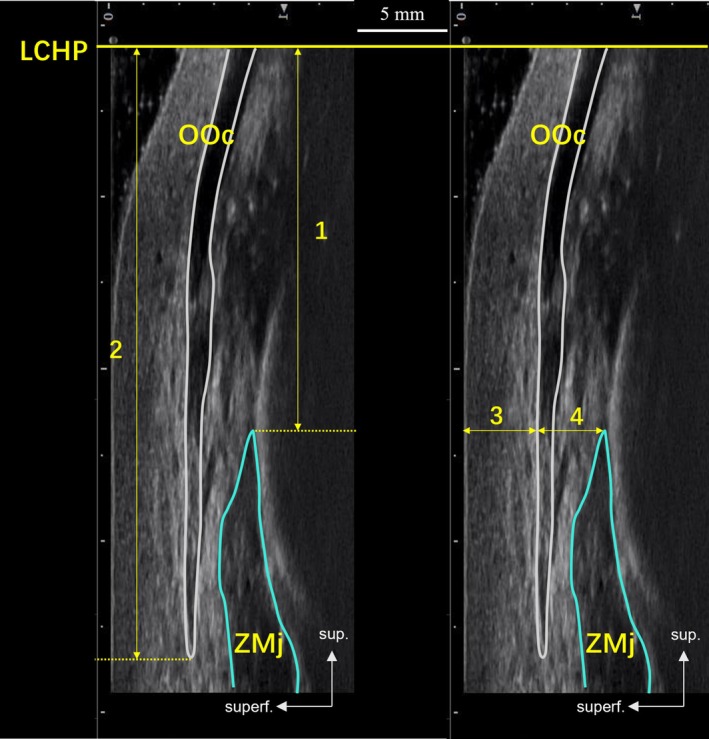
The 4 parameters measured on ultrasonographic examination of the orbicularis oculi muscle and the zygomaticus complex muscles. 1, the distance from the superior margin of the zygomaticus complex muscles to the lateral canthus horizontal plane (LCHP); 2, the distance from the inferior margin of the orbicularis oculi muscle (OOc) to the LCHP; 3, the distance from the epidermis to the surface of the OOc in the horizontal plane of the superior margin of the zygomaticus complex muscles; 4, the distance from the OOc to the surface of the zygomaticus complex muscles in the horizontal plane of the superior margin of the zygomaticus complex muscles. ZMj, zygomaticus major muscle.
